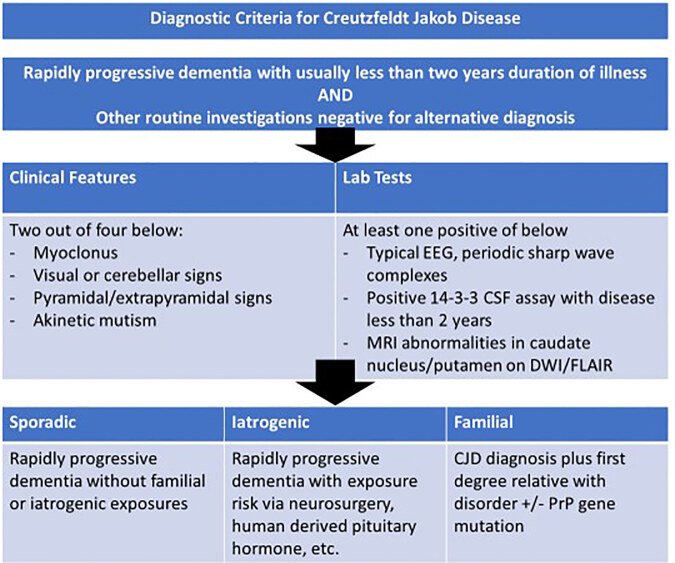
The diagnostic criteria for Creutzfeldt-Jakob disease.^9^ Amended from Centers for Disease Control and Prevention diagnostic criteria. *EEG*, electroencephalogram; *CSF*, cerebrospinal fluid; *MRI*, magnetic resonance imaging; *DWI*, diffusion-weighted imaging; *FLAIR*, fluid attenuated inversion recovery.
